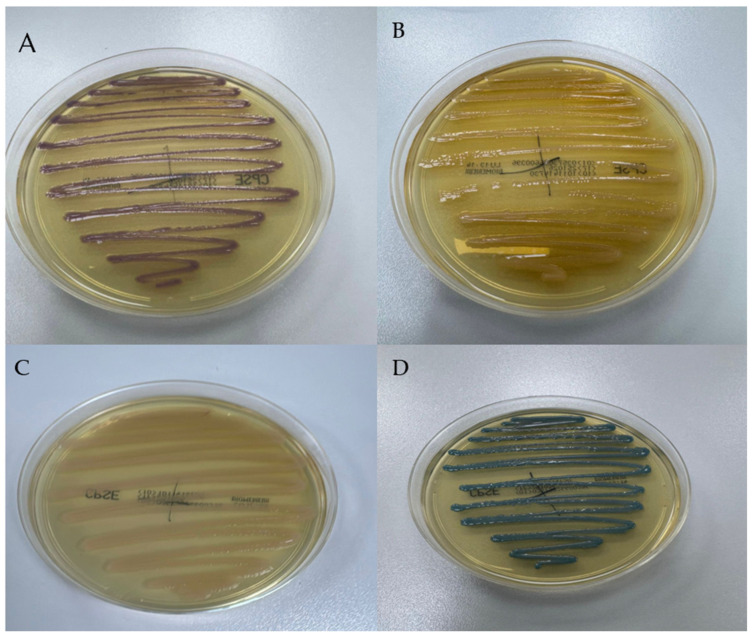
Colony morphology of uropathogens isolated from the urine of a 42-year-old male patient with non-Hodgkin large B-cell lymphoma, suspected primary immunodeficiency, and recurrent urinary tract infections, cultured on chromID CPS Elite medium (bioMérieux). Species identification was confirmed using Vitek 2 (bioMérieux): (A) Escherichia coli showing pink-to-burgundy colonies; (B) Proteus mirabilis producing light-beige colonies; (C) Pseudomonas aeruginosa with yellowish colonies; (D) Klebsiella/Enterobacter/Serratia group presenting green-to-blue colonies. Abdominal ultrasonography was performed during hospitalization in the nephrology ward, demonstrating normal renal size and morphology, with no evidence of hydronephrosis, lithiasis, or structural abnormalities of the urinary tract. The bladder wall appeared regular, with no post-void residual volume or mucosal thickening, effectively excluding obstructive or anatomic causes for recurrent infections. A contrast-enhanced CT scan of the abdomen and pelvis confirmed the absence of congenital or structural urinary tract anomalies. The patient had no history of voiding dysfunction or neurogenic bladder. Urodynamic studies and cystoscopy were therefore not indicated.
